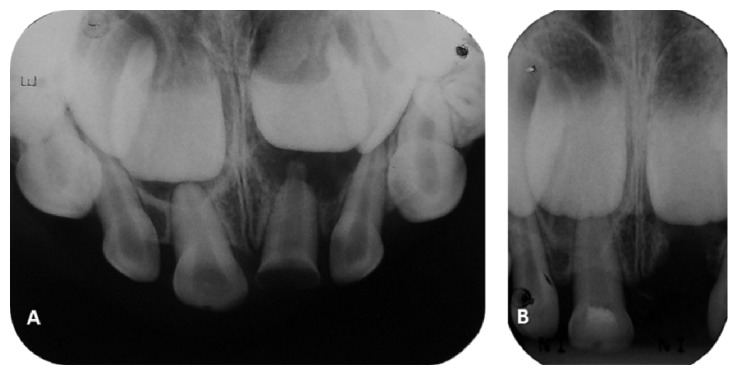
(A) The initial periapical radiography showing no periapical alterations in (51) and lesion in (61) with disruption of the dental follicle of the permanent left maxillary central incisor (21). (B) The periapical radiography after the pulpotomy of tooth (51) and the extraction of (61).
